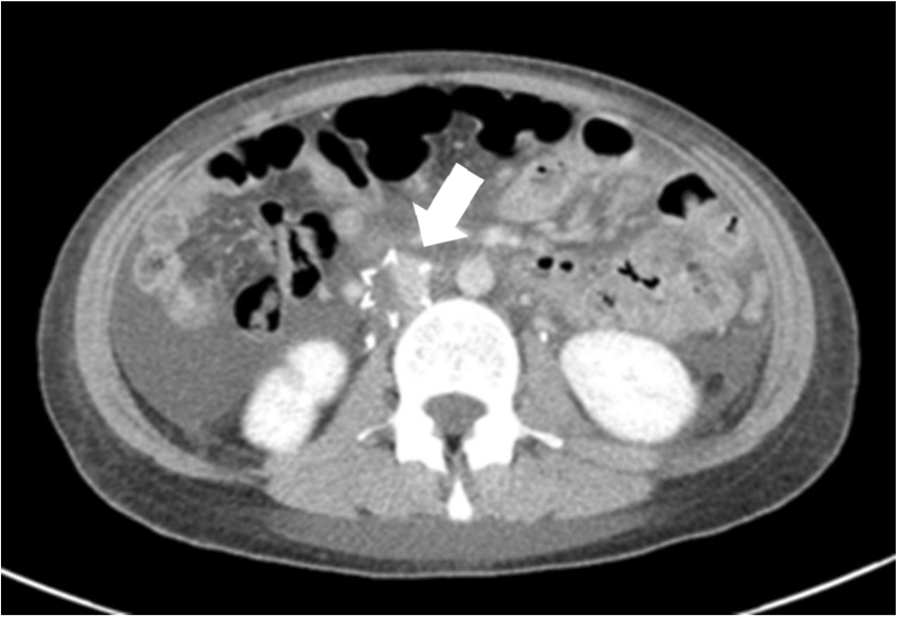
Computed tomography taken at day 14 showing capture of thrombi in the inferior vena cava filter (arrow).
